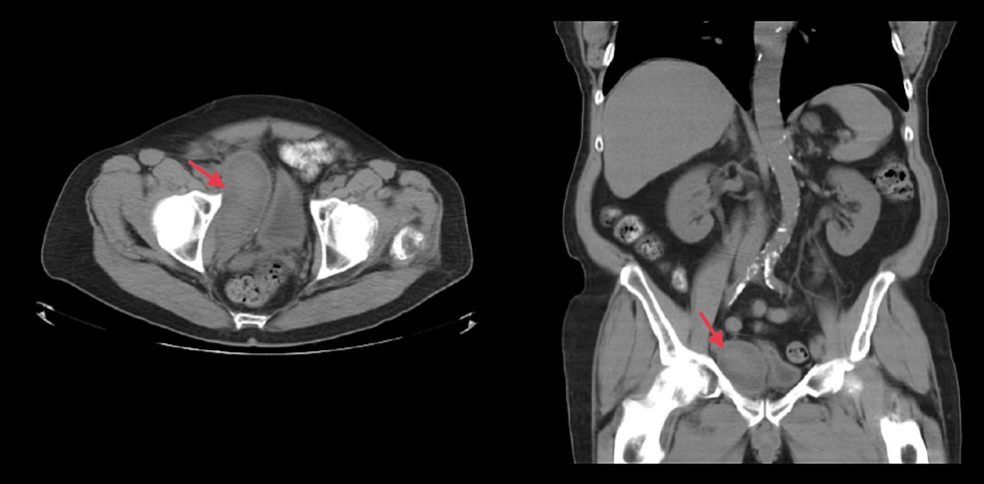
Pelvic CT scan eight weeks after UroLift showing a right pelvic soft tissue density mass with central low attenuation measuring 10.4 cm x 4.5 cm x 5.0 cm in diameter exerting a mass effect on the urinary bladder consistent with a pelvic hematoma.
